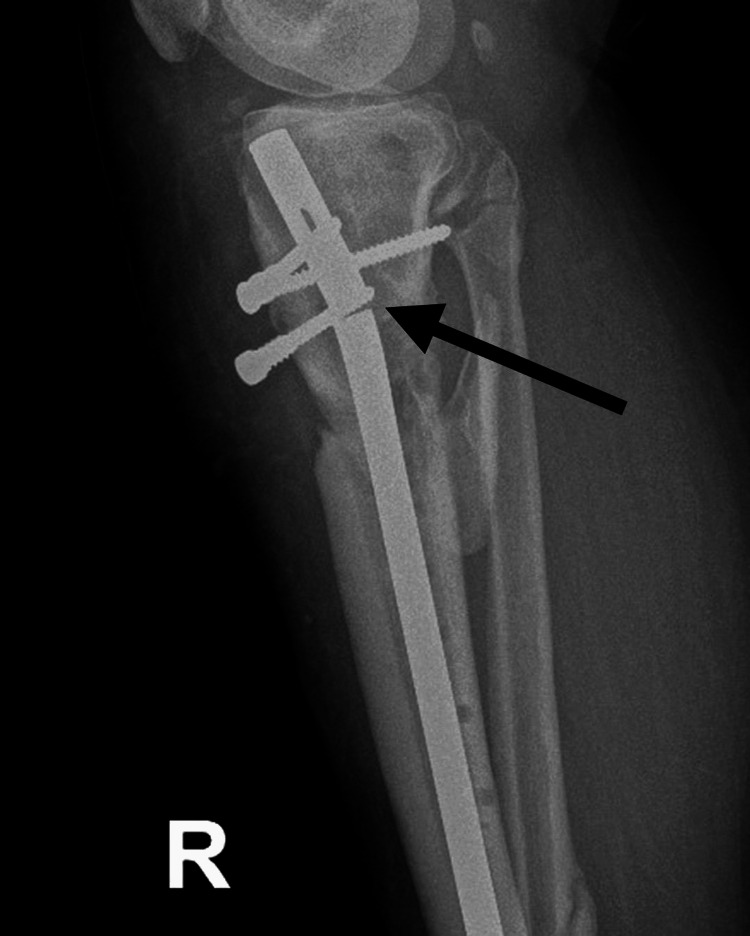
Lateral X-ray seven months after the second surgical intervention, showing oligotrophic nonunion and implant failure (arrow points to the implant failure)
